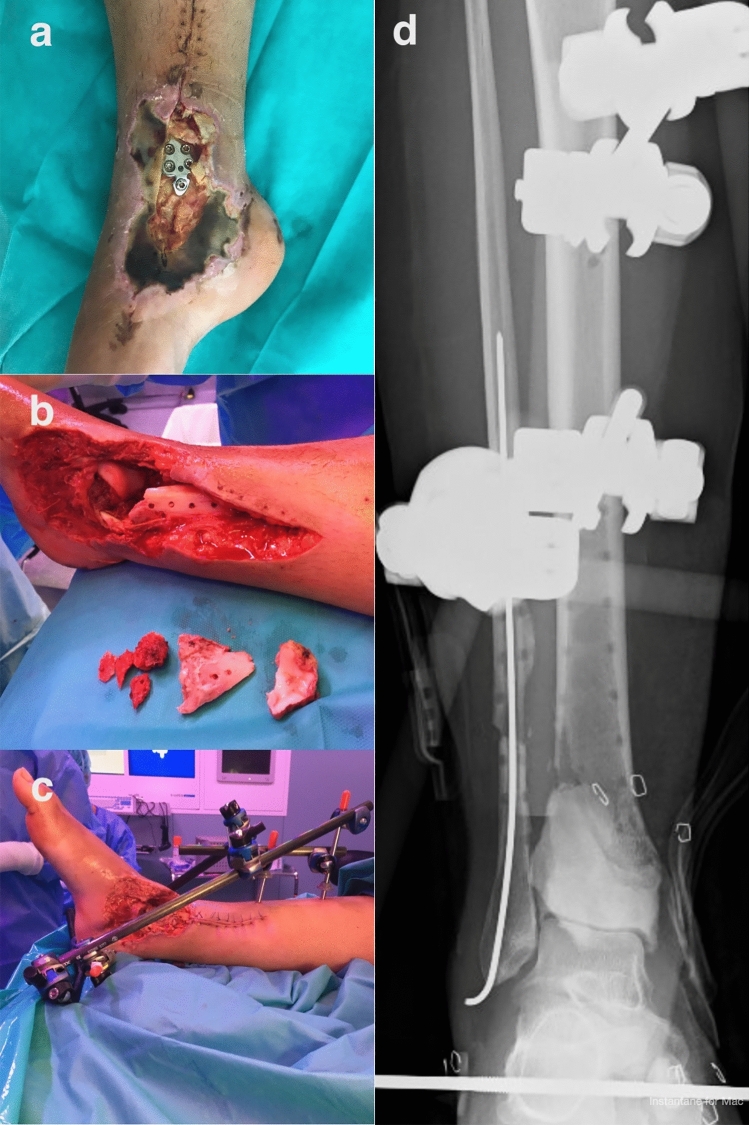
Panel of images demonstrating the 3-stage protocol for management of an infected distal tibial fracture initially stabilized with a plate; a preoperative clinical image of a 32-year-old woman with a massive infected soft-tissue defect and a long segment of grossly necrotic bone (with joint involvement); b intra-operative image following radical debridement, resulting in a critical segmental bone defect (5 cm) now replaced with antibiotic-laden PMMA; c intra-operative image demonstrating a cement spacer together with a temporary (< 10 days) modular external fixator used to obliterate dead space and confer stability; d post-operative radiograph after completing the first stage; negative pressure wound therapy (NPWT) device applied to isolate the wound between first and second stages
